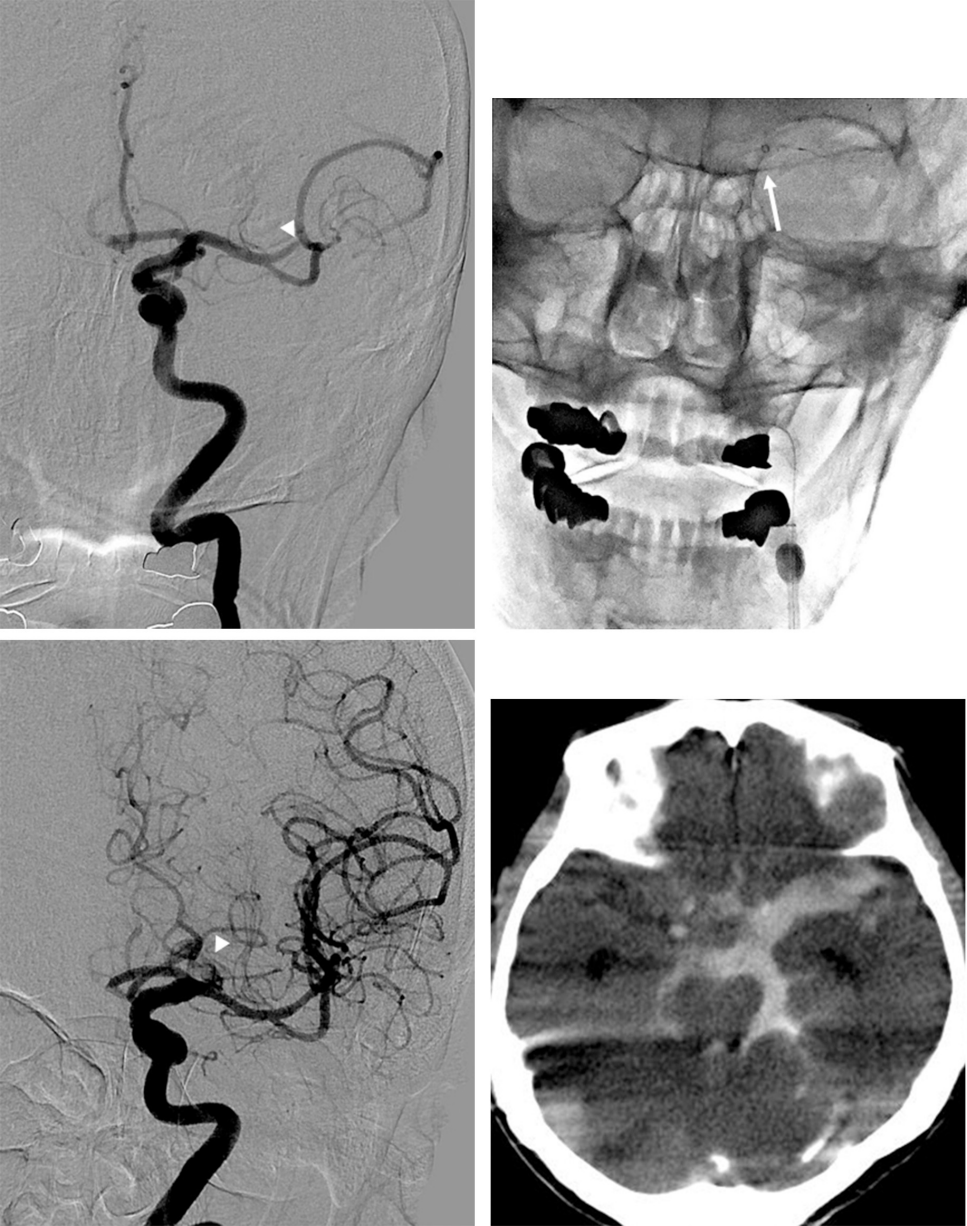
An 87-year-old man, who presented with acute stroke and an initial NIHSS score of 18. (A) Left anteroposterior internal carotid artery angiogram shows occlusion of the proximal superior trunk of the middle cerebral artery (arrowhead). (B) The advancement of the distal access catheter (DAC) in the middle cerebral artery was very difficult. The DAC was advanced in the proximal middle cerebral artery (arrow). (C) Post-thrombectomy anteroposterior internal carotid artery angiogram shows complete reperfusion of the middle cerebral artery; however, there is contrast extravasation (arrowhead) in the left proximal middle cerebral artery. (D) Postoperative axial CT shows subarachnoid hemorrhage.
